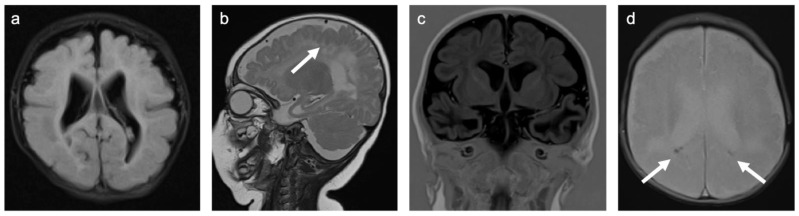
Representative case “Infectious white matter damage”: congenital cytomegalovirus infection. FLAIR (a), T2W (b), Turbo inversion recovery (TIR; (c)), and T2*W (d) sequences. Four-month-old girl with a history of congenital cytomegalovirus infection. MRI shows microcephaly with polymicrogyria ((b); white arrow), bilateral ventriculomegaly (a–d), global thinning of the cerebral mantle, especially temporal (b,c), bilateral white matter signal abnormalities in the frontal and parieto-occipital regions (a), and punctuate periventricular calcifications ((d); white arrows).
